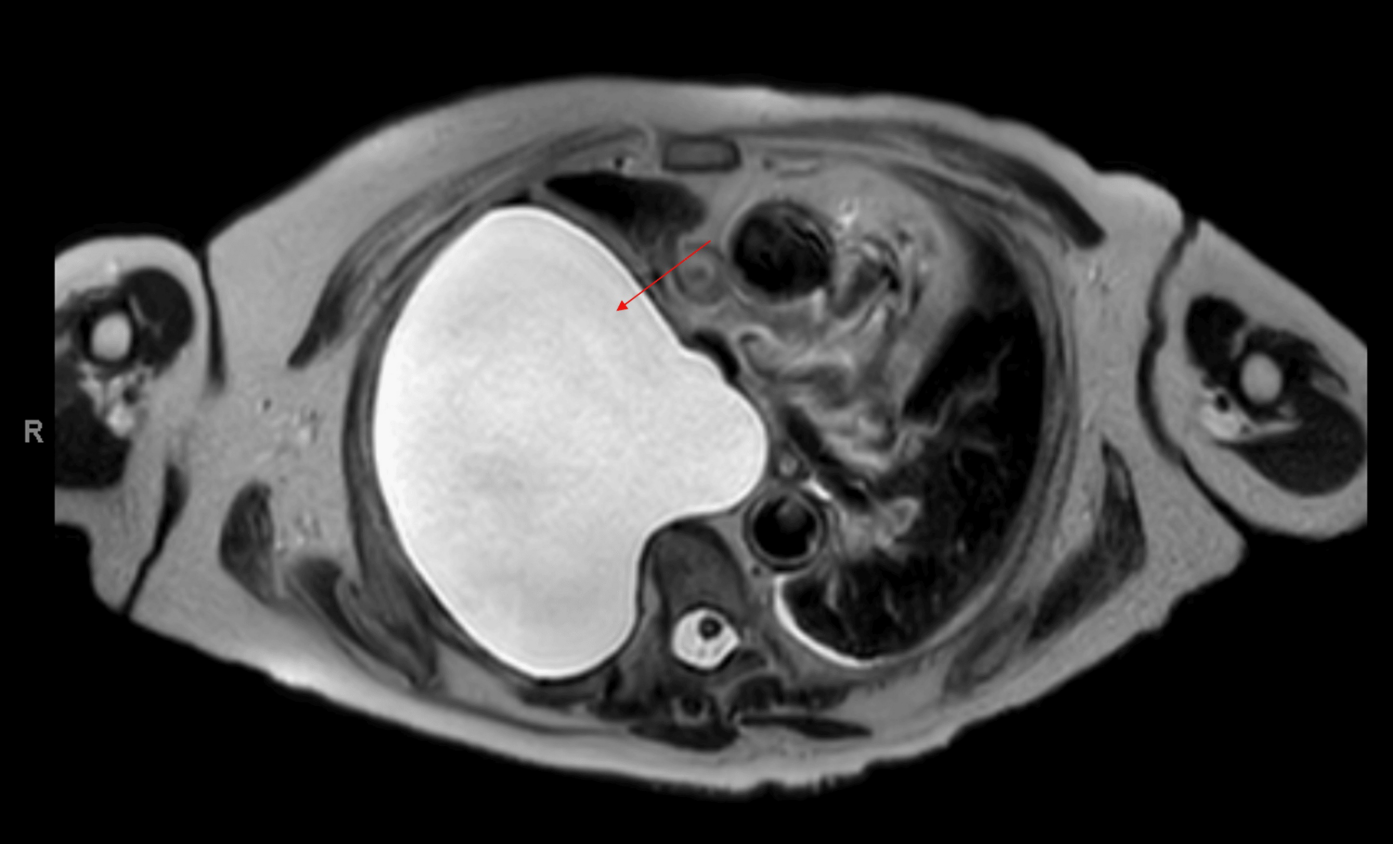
Axial T2 weighted MRI image
